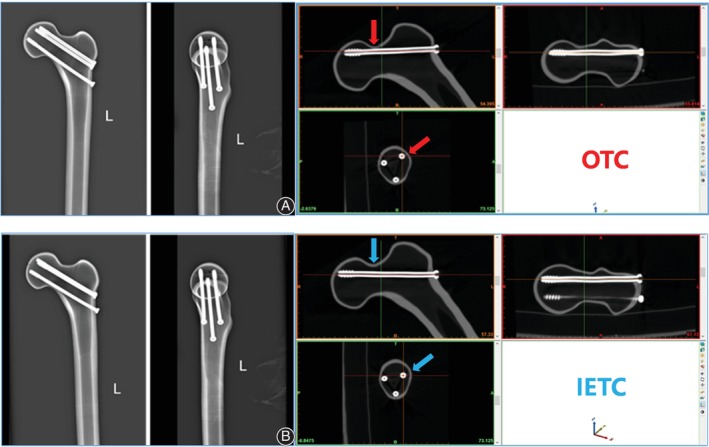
The anteroposterior and lateral X‐ray and CT images of a fixed femoral neck fracture model with CCSs in two configurations. (A) OTC. (B) IETC. The position of the femoral calcar screw in the two configurations was consistent. The OTC position of the posterior screw was slightly higher than that of the IET (indicated by the red and blue arrows respectively), and the OTC position of the anterior screw was significantly higher than that of the IETC. In OTC, three screws were attached to the femoral neck cortex, while in IETC, the anterior and superior screws were not attached to the femoral neck cortex, and did not achieve cortical support.
